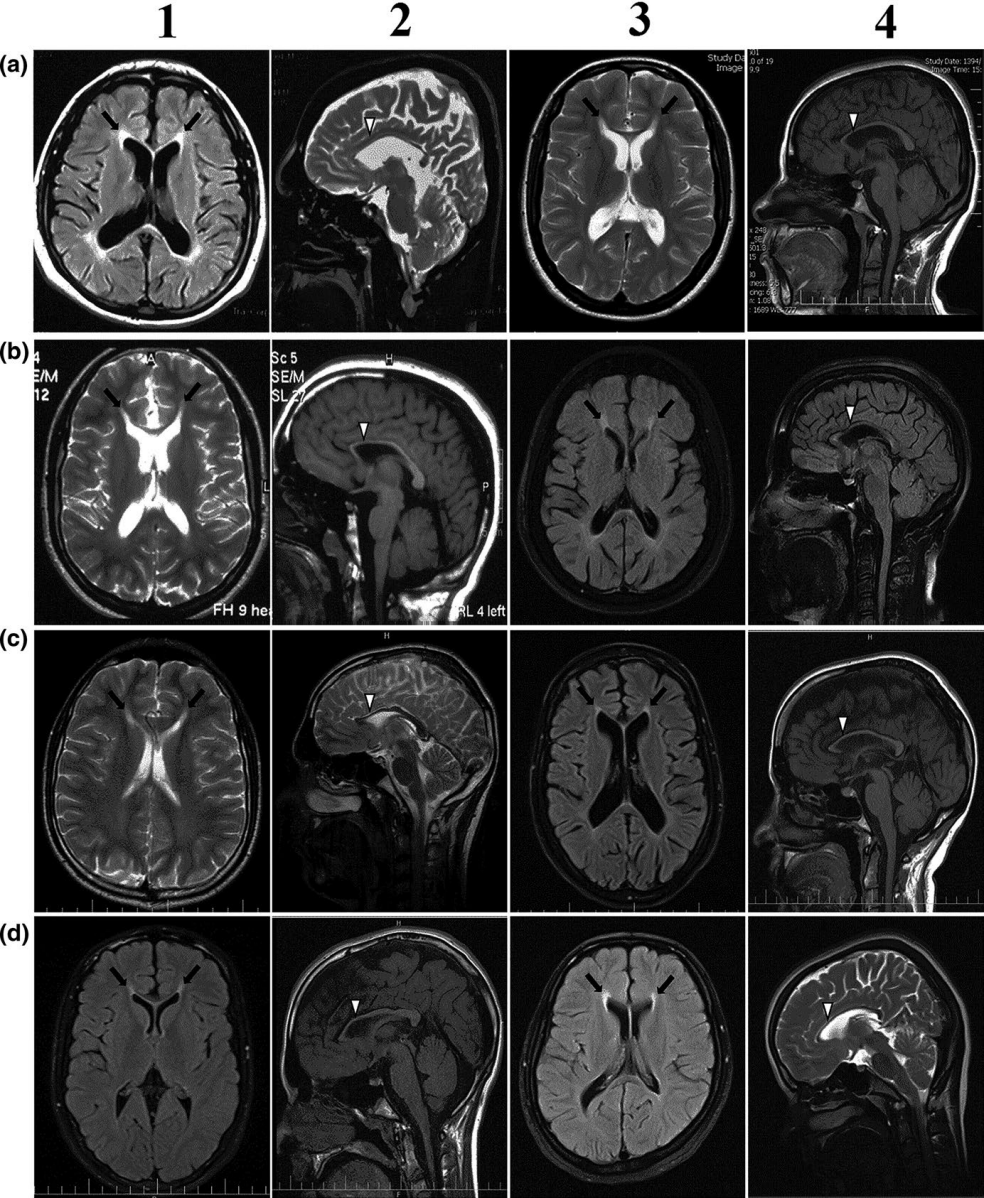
Representative brain MRI images of patients with *SPG11* mutations. a1 and a2, SPG11‐300‐II1; a3 and a4, SPG11‐102‐II3; b1 and b2, SPG11‐102‐II4; b3 and b4, SPG11‐101‐II3; c1 and c2, SPG11‐101‐II7; c3 and c4, SPG11‐189‐II2; d1 and d2, SPG11‐189‐II3; d3 and d4, SPG11‐210‐II7. Rows 1 and 3, axial views; rows 2 and 4, sagittal views. Hyperintensities, which are more prominent in anterior regions next to frontal horns (black arrows), are evident in the axial images. Thin corpus callosum, which is significantly more prominent in anterior/rostral parts (white arrow heads), is shown in the sagittal images
